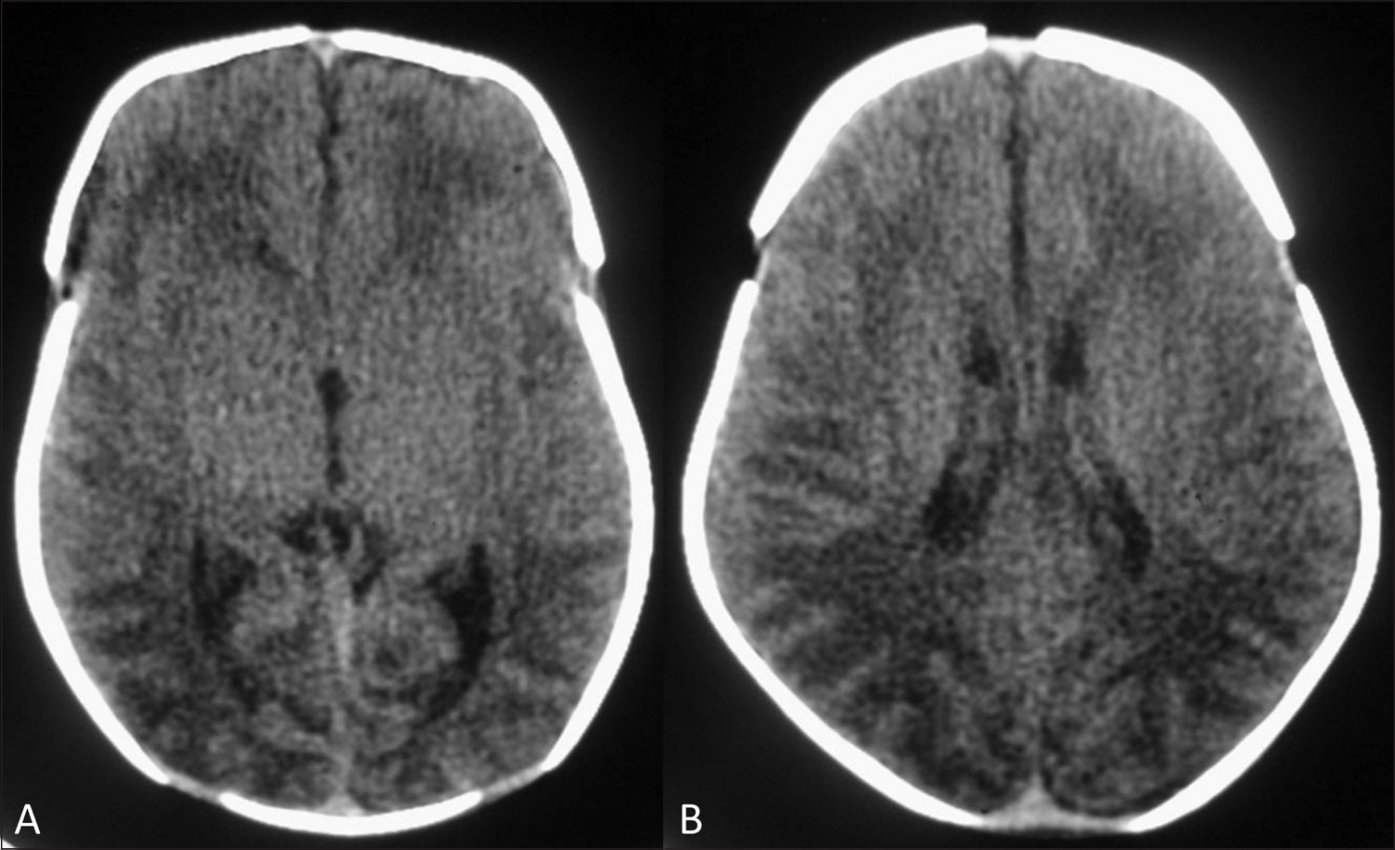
Neonatal hypoglycemia: CT scan of the brain shows typical low attenuation in the white matter of the occipital (A) and parietal (B) lobes on both sides
